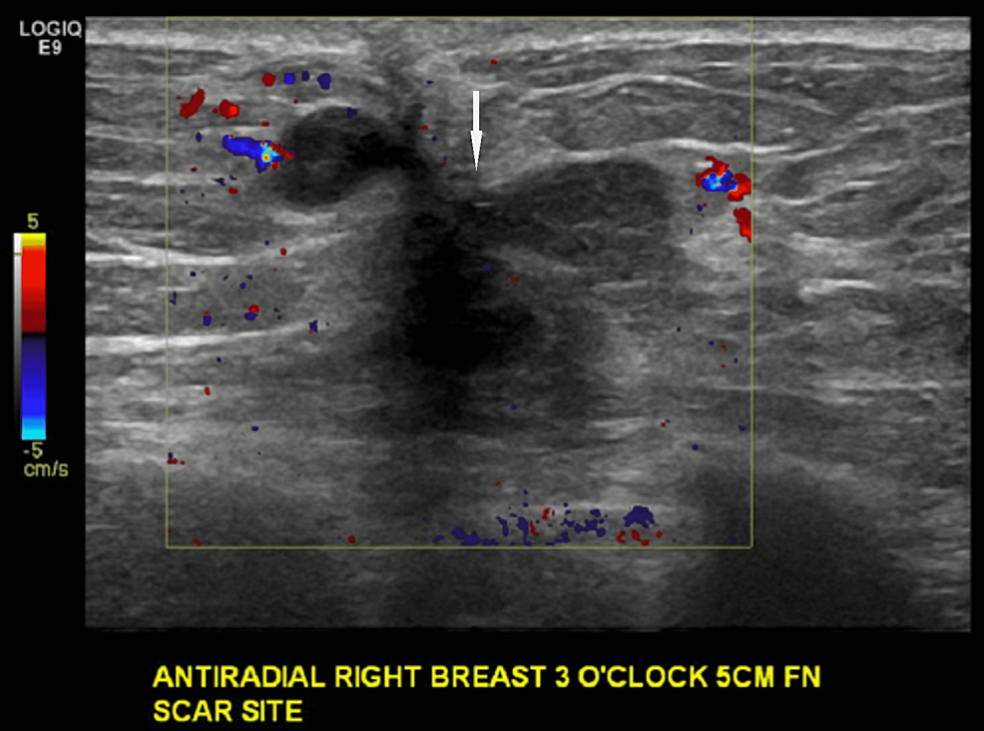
Targeted ultrasound of the right breast at the site of mammographic finding and surgical scar at the time of presentation. Corresponding to the mammographic finding of new increasing density at the site of the scar, there is an irregular, hypoechoic, shadowing mass at three o'clock 5 cm from the nipple.
